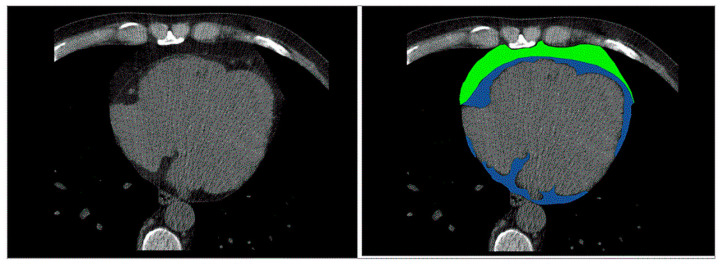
Cardiac Computed Tomography: EAT (depicted in blue) is located between the myocardium and visceral pericardium, PAT (depicted in green) is located adherent and external to the parietal pericardium. EAT: epicardial adipose tissue; PAT: pericardial tissue. de Wit-Verheggen VHW, et al. Cardiovasc Diabetol. 2020;19:129, under Creative Commons license 4.0.
